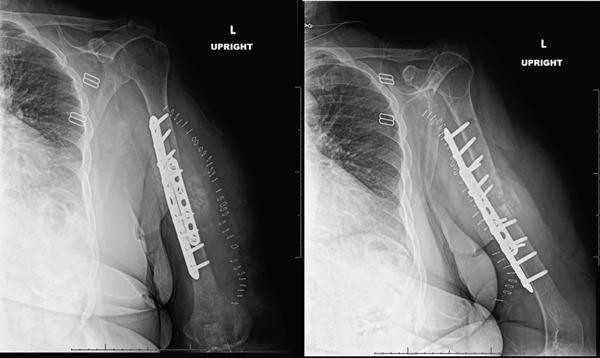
Radiographs obtained 3‐weeks postoperative demonstrating hardware failure with the large frag plate pulling out of the proximal segment and two screws pulling out of the small frag plate. There is some callus formation posteriorly. Overall, the alignment of the humerus was maintained.
